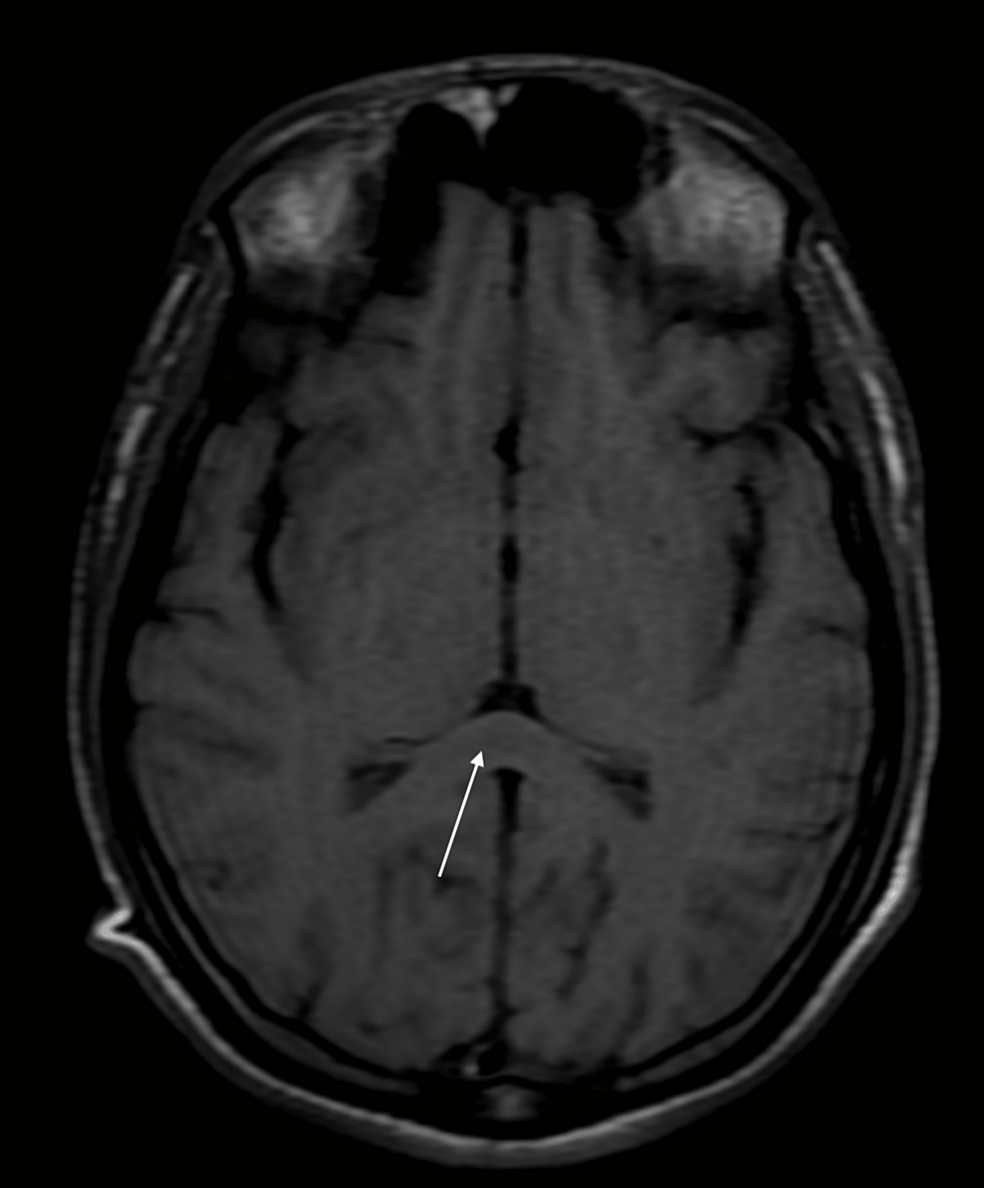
MRI imaging showing hypointense signal on the T1WI T1WI: T1-weighted
